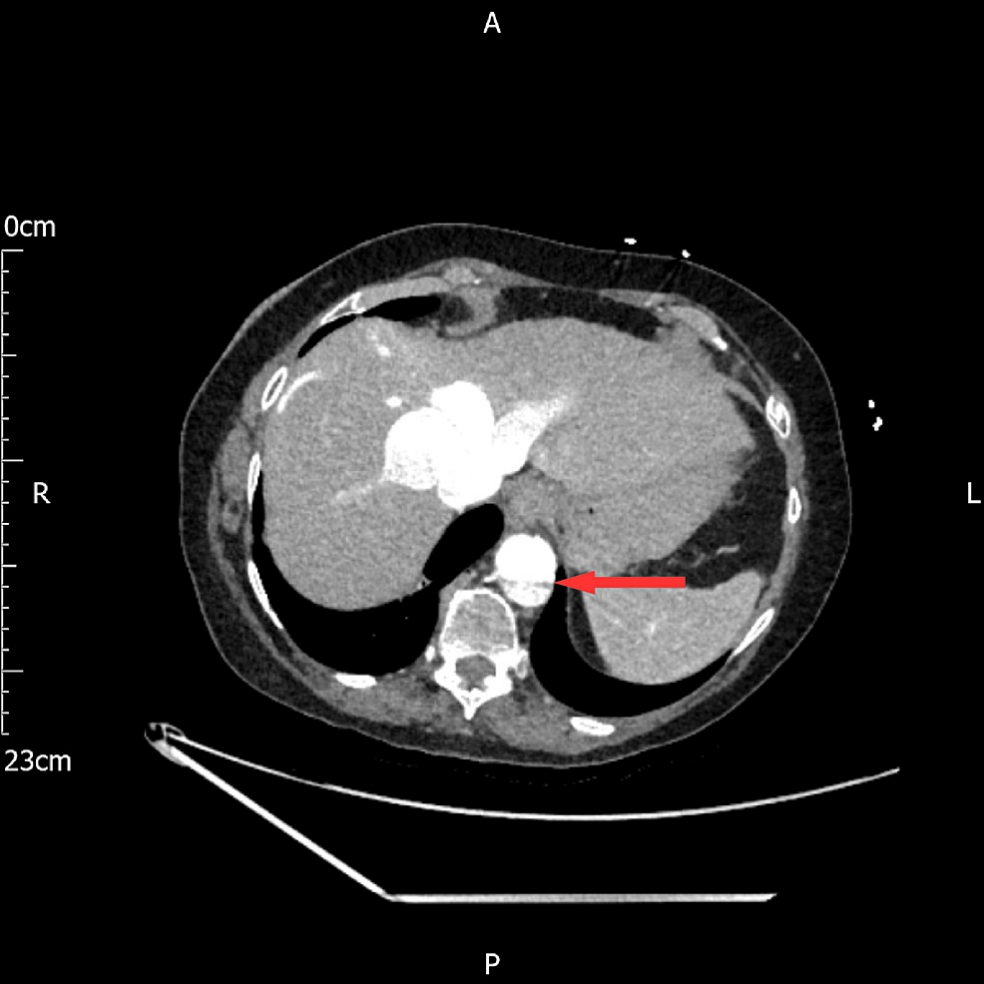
Arterial phase CTA showing aortic dissection at the T 10 level CTA; computed tomography angiogram, T; thoracic
